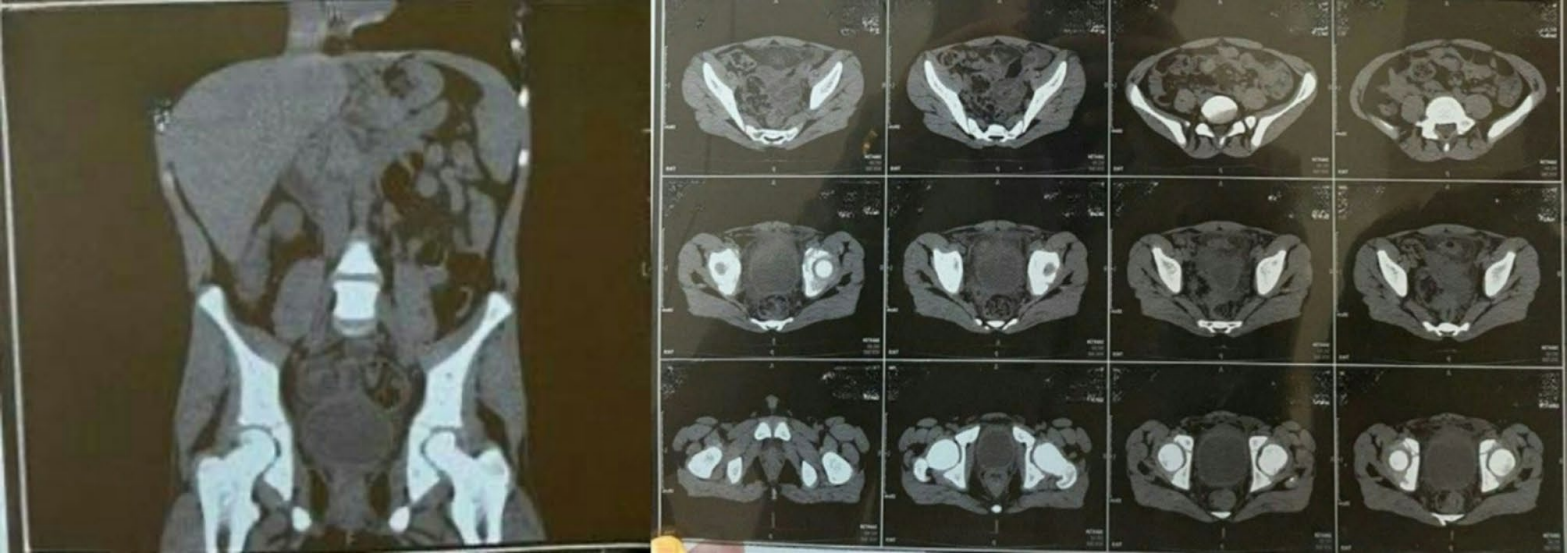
Non-contrast spiral (multi-slice) computed tomography (CT) scan of the abdomen and pelvis revealed a thickened blind-ended structure in the midline of the lower abdomen anterior to urinary bladder It shows a gas focus inside with extensive stranding of surrounding fat planes
